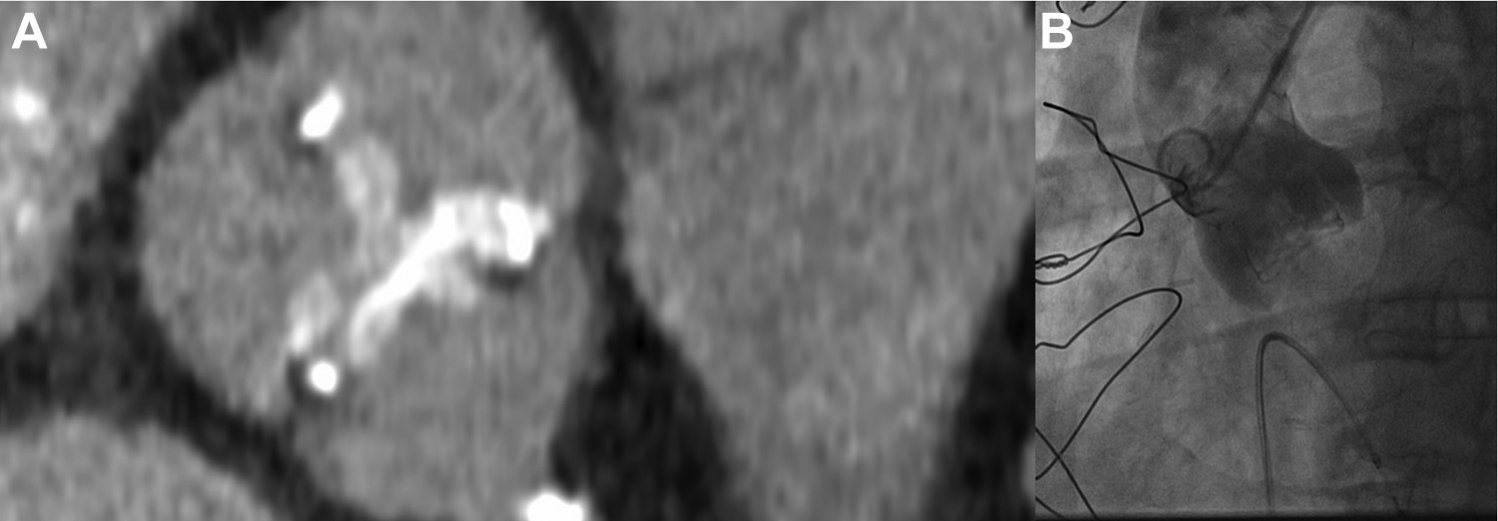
Computed Tomography and Angiographic Images Showing Relationship Between Coronary Ostia, Surgical Bioprosthesis and Aorta (A) Transverse plane at the level of the post of the aortic bioprosthesis obtained from the angiographic computed tomography. Image shows a correct alignment of the post of surgical valve and coronary ostium. The distance between coronary ostia and valve was >9 mm, indicative of a low probability of coronary obstruction during valve-in-valve intervention. (B) Aortography performed before the valve-in-valve procedure.
